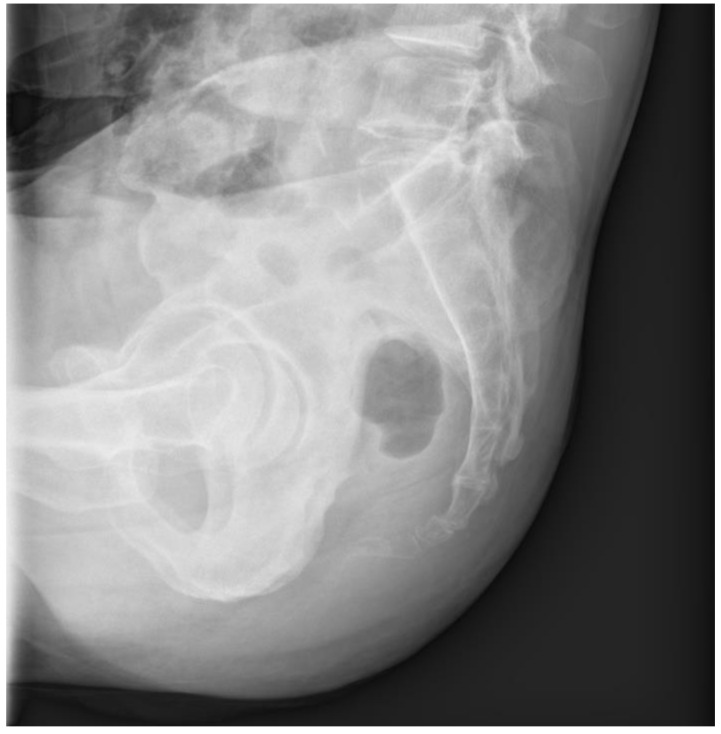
Plain lateral radiography performed six months after the operation. The sacrococcygeal joint maintained good alignment.
